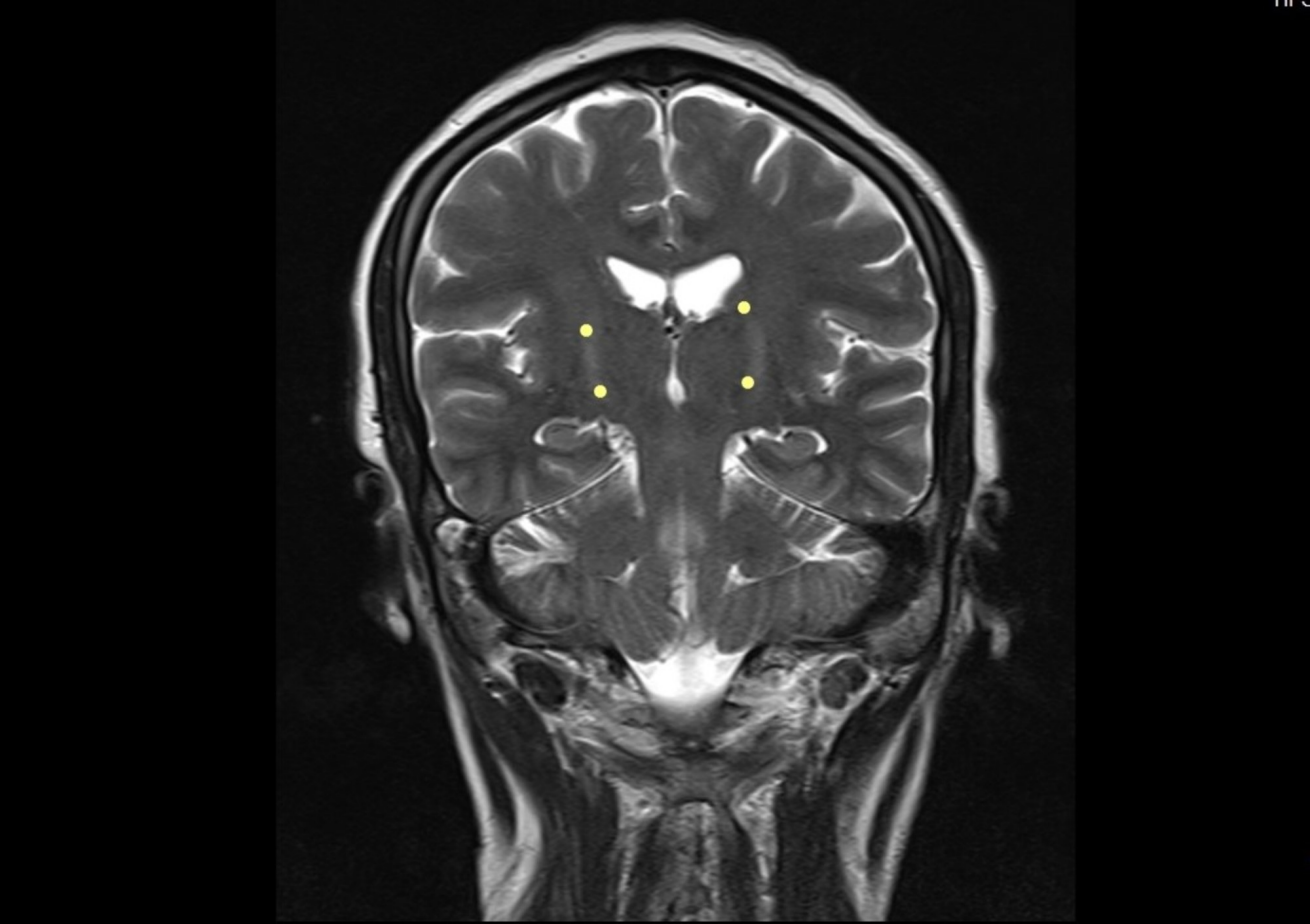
Brain MRI - view 4 FLAIR axial and T2-weighted coronal image. The image shows hyperintensity in the path of the corticospinal tract (yellow dots) MRI: magnetic resonance imaging; FLAIR: fluid attenuation inversion recovery
